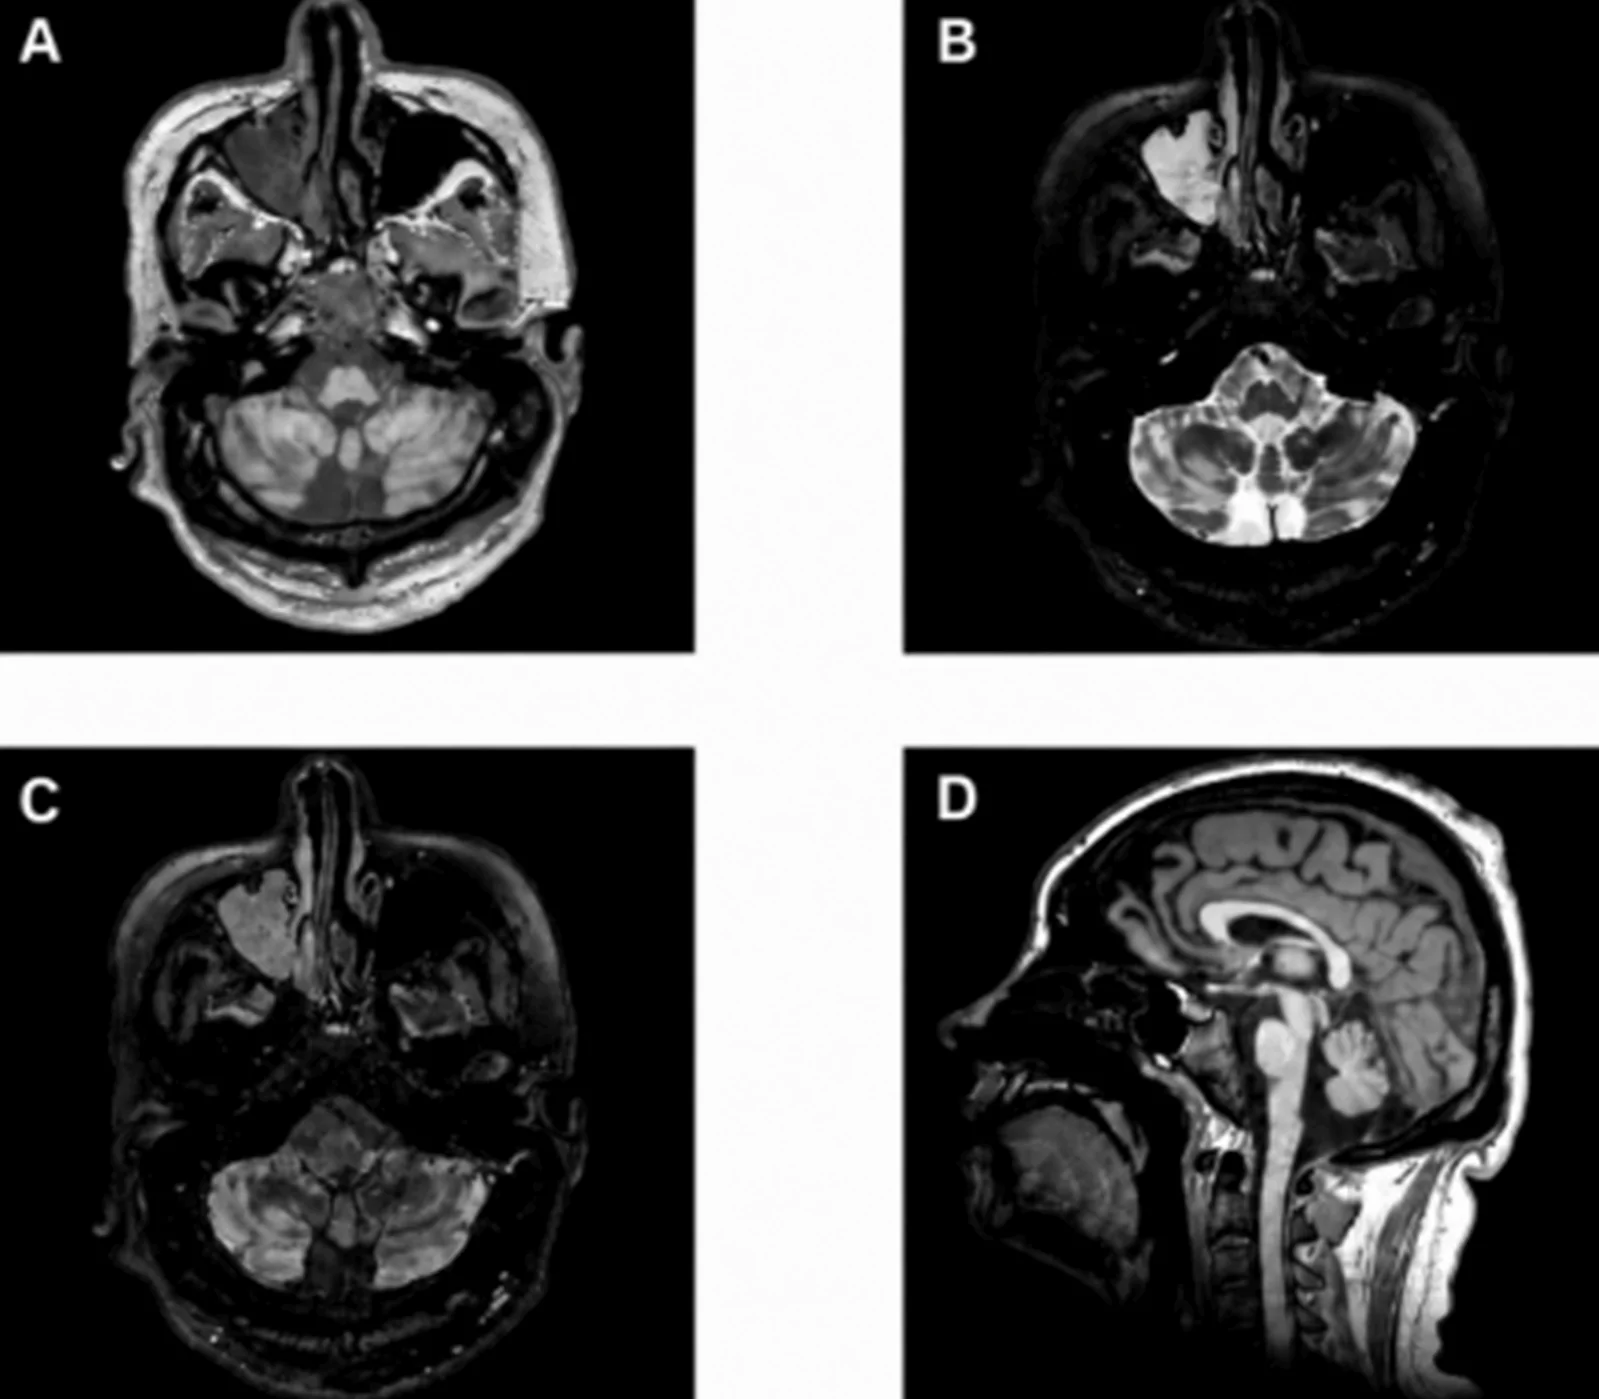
Brain magnetic resonance imaging. (A) Axial T1-weighted image showing a bilateral, relatively symmetric abnormality of the cerebellar hemispheres in the region of the ansiform lobule, characterized by decreased signal intensity. (B) Axial T2-weighted image showing bilateral hyperintensity in the same anatomical distribution and blurring of the gray-white matter differentiation. (C) Axial fluid-attenuated inversion recovery (FLAIR) image confirming bilateral hyperintensity predominantly involving the lateral and posterior cerebellum. (D) Midline sagittal T1-weighted image showing relative sparing of the cerebellar vermis.
